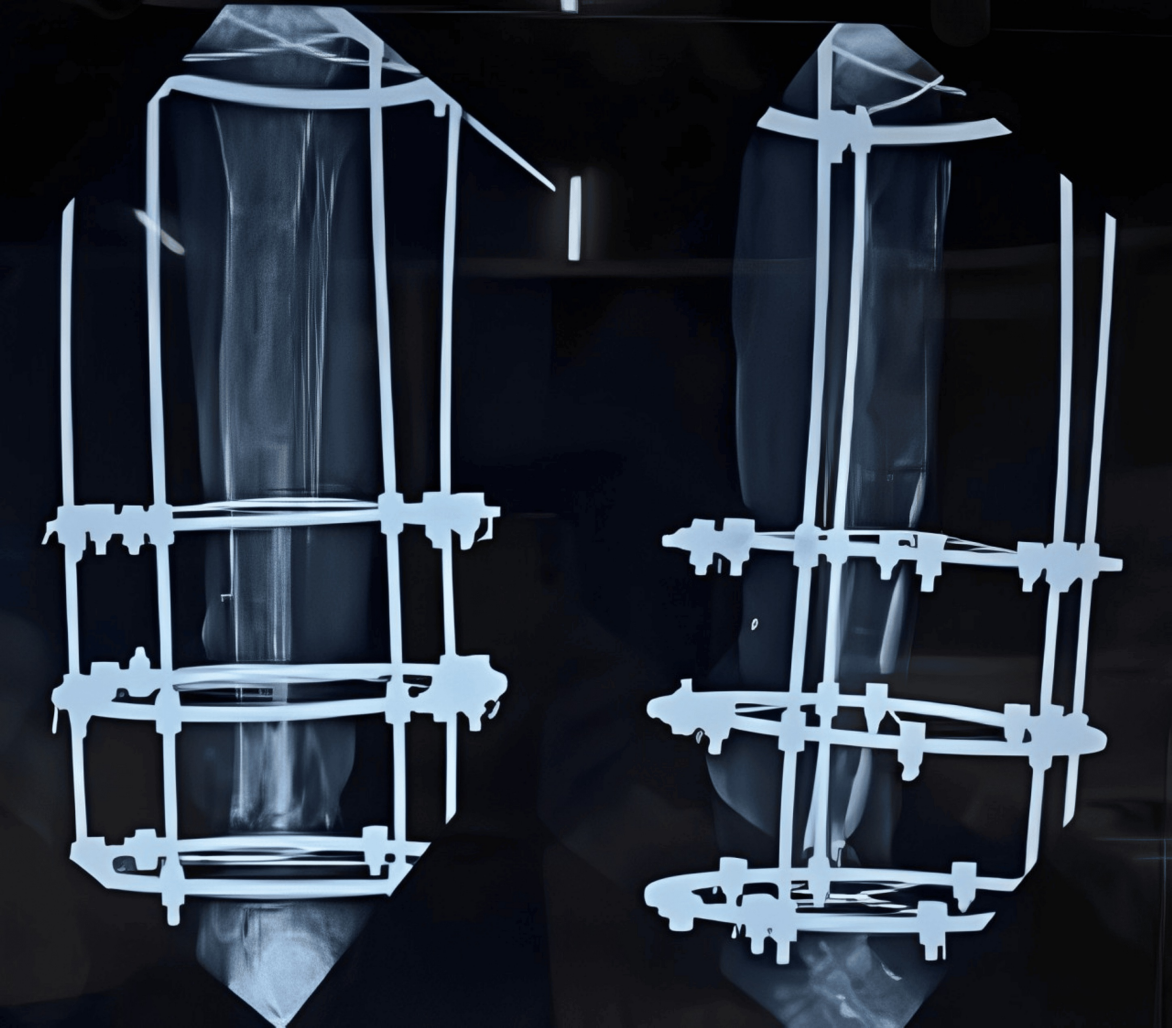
X-ray showing the consolidation and healing of the distraction site (after three months of stopping the distraction)
